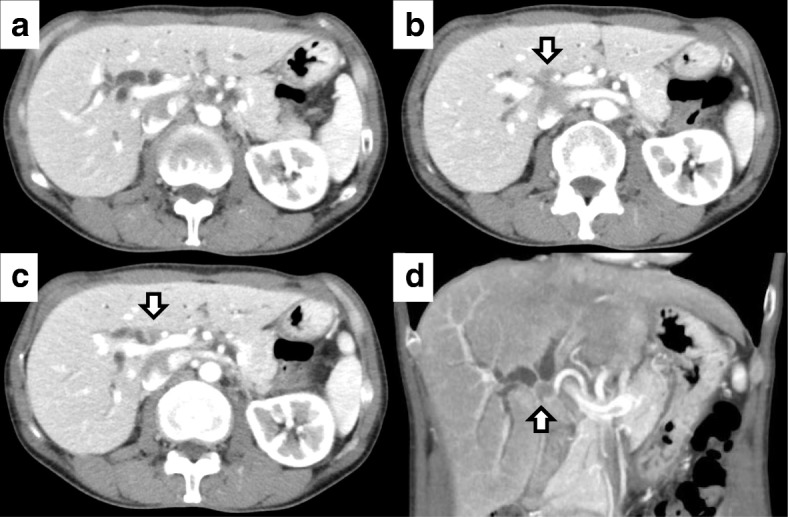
Contrast-enhanced abdominal computed tomography (CT) findings. Contrast-enhanced CT showed an approximately 10-mm high-attenuation intraductal mass at the hepatic hilum (arrow) and dilation of the intrahepatic bile duct. a–c Axial views. d Coronal view
